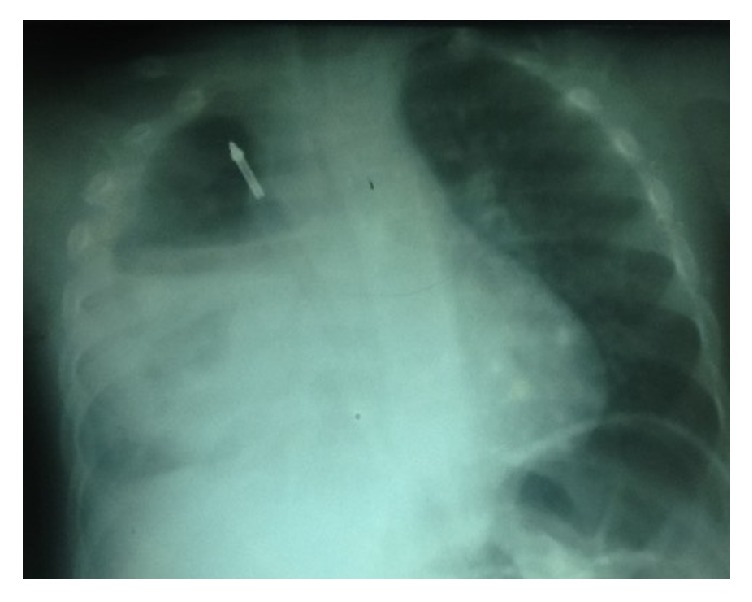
Chest X-ray one month later. This picture was taken the day of his arrival at our hospital. The empyema is loculated, the foreign body is still present in the chest, and the radiological signs of esophageal perforation are more obvious.
